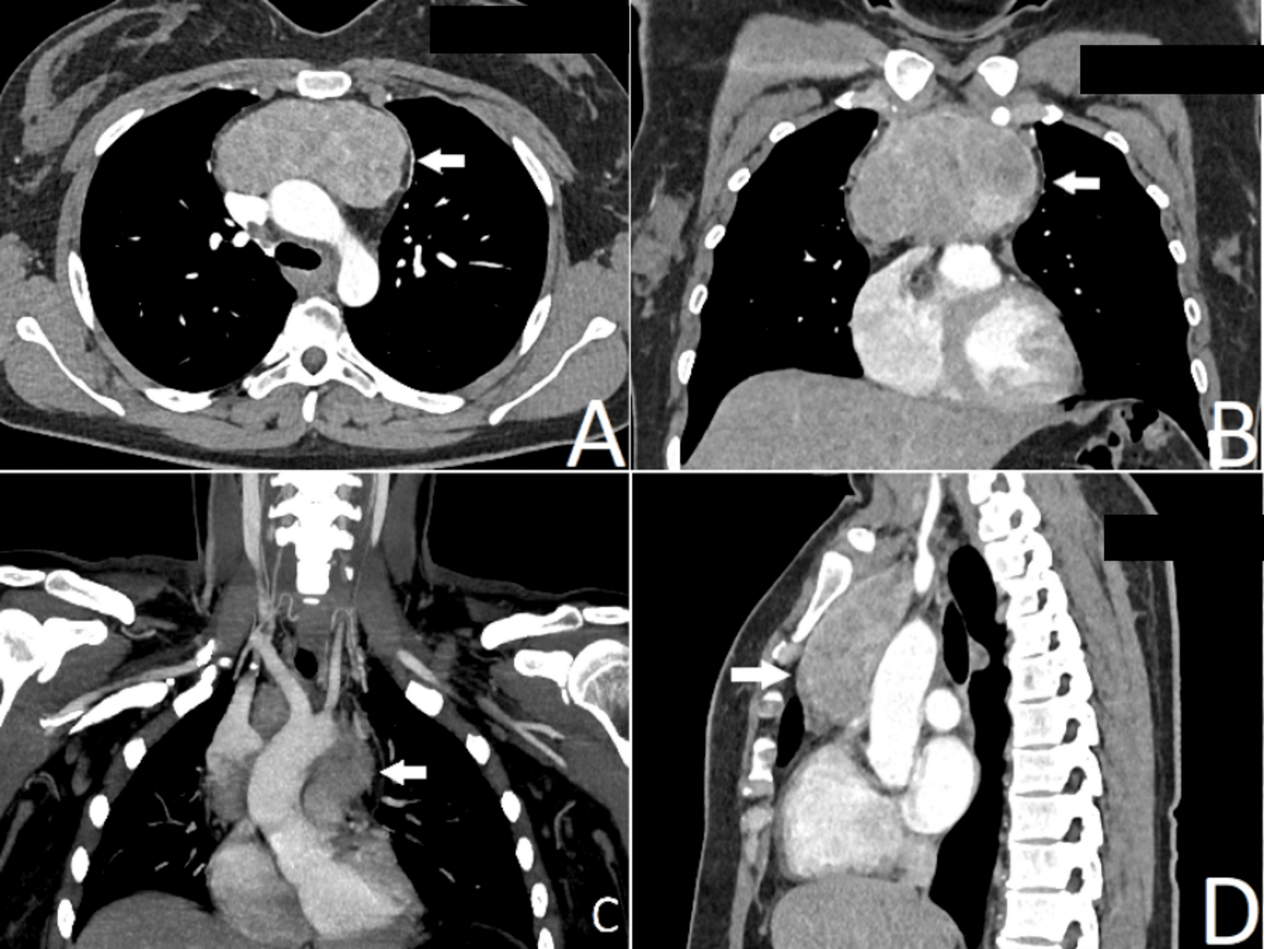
Contrasted computed tomography, showing a retrosternal goiter (white arrows).
